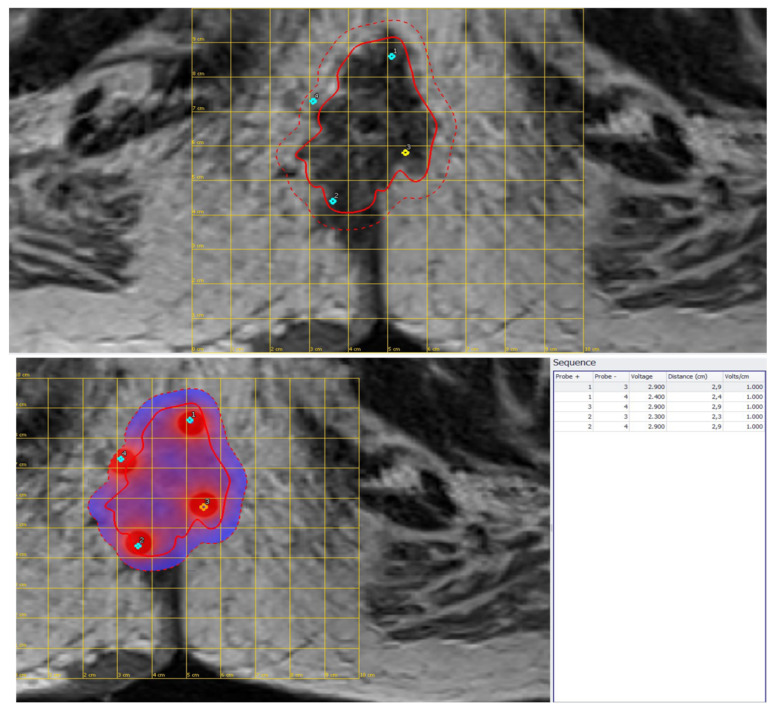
Preoperative planning of third LARC case effected using MR images.
